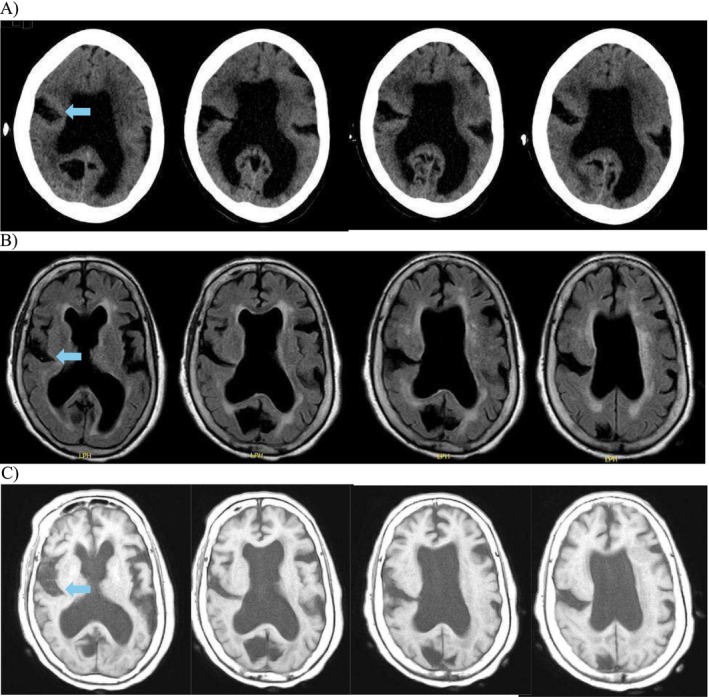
(A) Computerized tomography brain without contrast showing inferior to superior sequence (shown left to right) of right‐sided schizencephaly extending from the cortex to the right lateral ventricle. (B) Magnetic resonance imaging brain without contrast (axial T2 FLAIR) showing inferior to superior sequence (shown left to right) of right‐sided schizencephaly extending from the cortex to lateral ventricle and hypoplasia of the corpus callosum. (C) Magnetic resonance imaging brain without contrast (axial T1) showing margins of the schizencephaly defect lined with dysplastic gray matter. *Arrows indicate cleft extending from the right cerebral cortex into the lateral ventricle.
